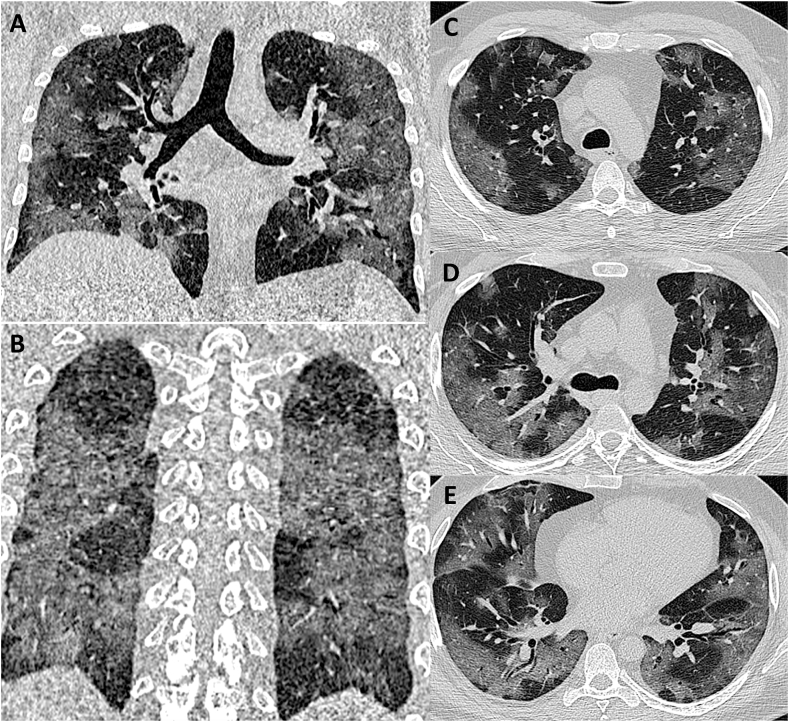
Noncontrast chest CT images of a 44-year-old man. Coronal reformatted (A and B), and axial (C–E) images show multiple ground glass opacities in multiple lung segments and a CT-SS of 32.
